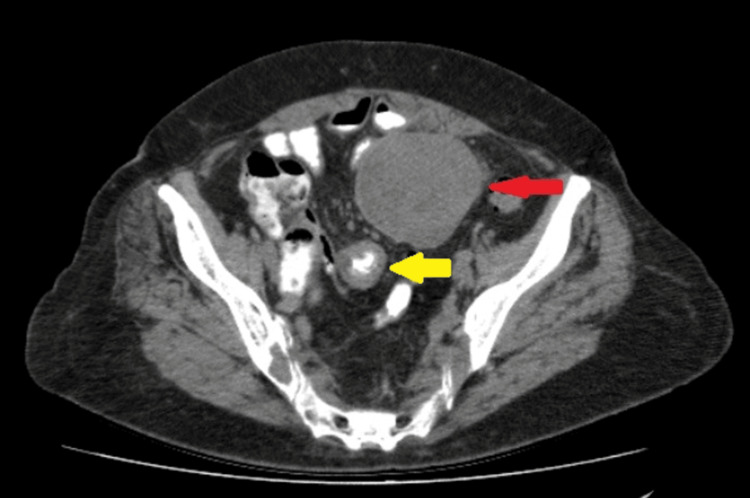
The CT scan (transverse view) showing a lesion (red arrow) and bowel wall thickening (yellow arrow).
